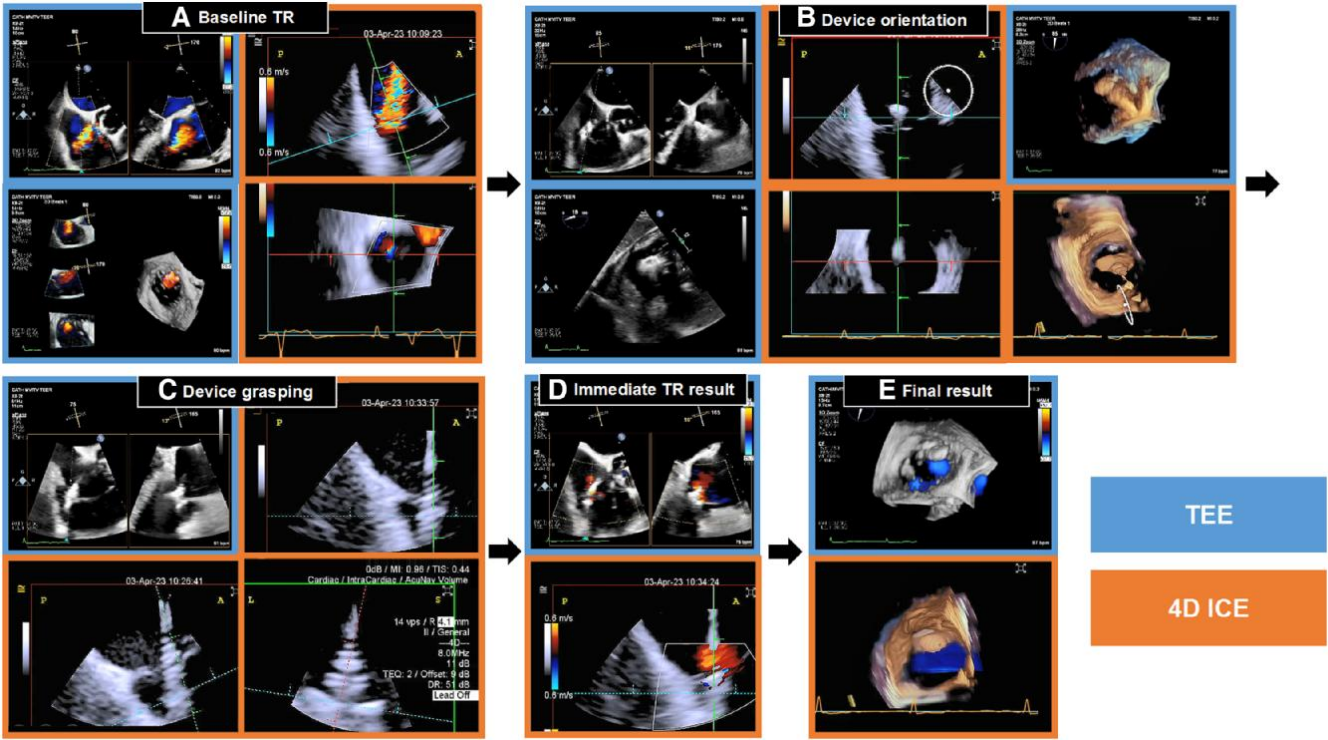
Transoesophageal echocardiography (TEE) is considered the standard imaging guidance tool for tricuspid transcatheter edge-to-edge repair (TEER). Common limitations of TEE imaging of the tricuspid valve (TV) include lower far-field spatial resolution and acoustic shadows stemming from calcific structures and metal containing intracardiac devices. The tricuspid leaflets may be particularly challenging to visualize during tricuspid TEER procedures. Intracardiac echocardiography (ICE) may overcome these intrinsic limitations.
